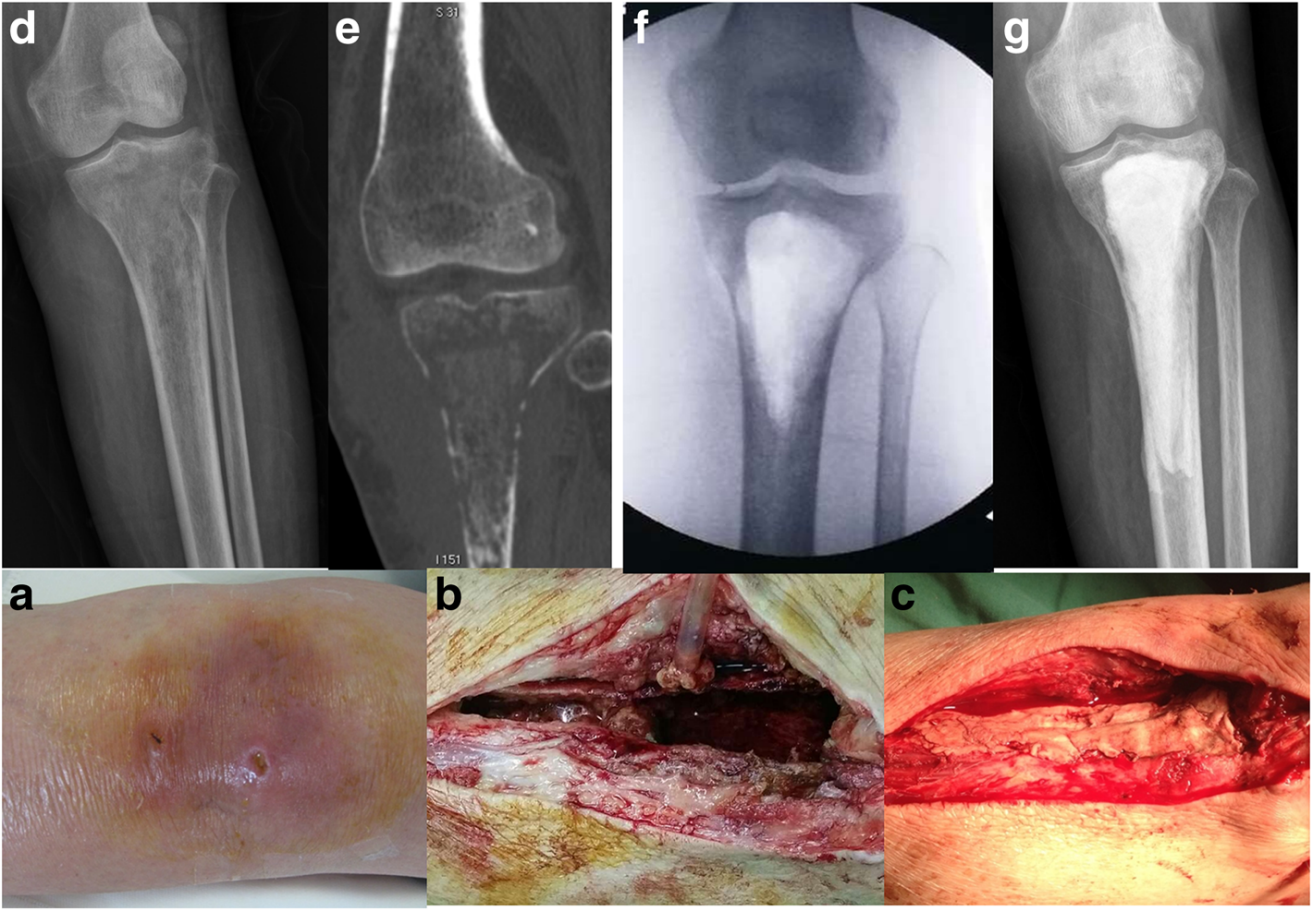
Case 3 (female, 70 years old) required long-term glucocorticoid treatment because of interstitial pneumonia. She suffered from left tibia osteomyelitis and failed treatment with intravenous antibiotic therapy. a Tenderness, warmth and effusion were observed on admission one month after the onset of osteomyelitis. d-e X-ray film and CT scan revealed bone destruction on admission. b, f hrough debridement was performed; a significant bone defect occurred after debridement. c, g The bone defect was filled with bone cement
